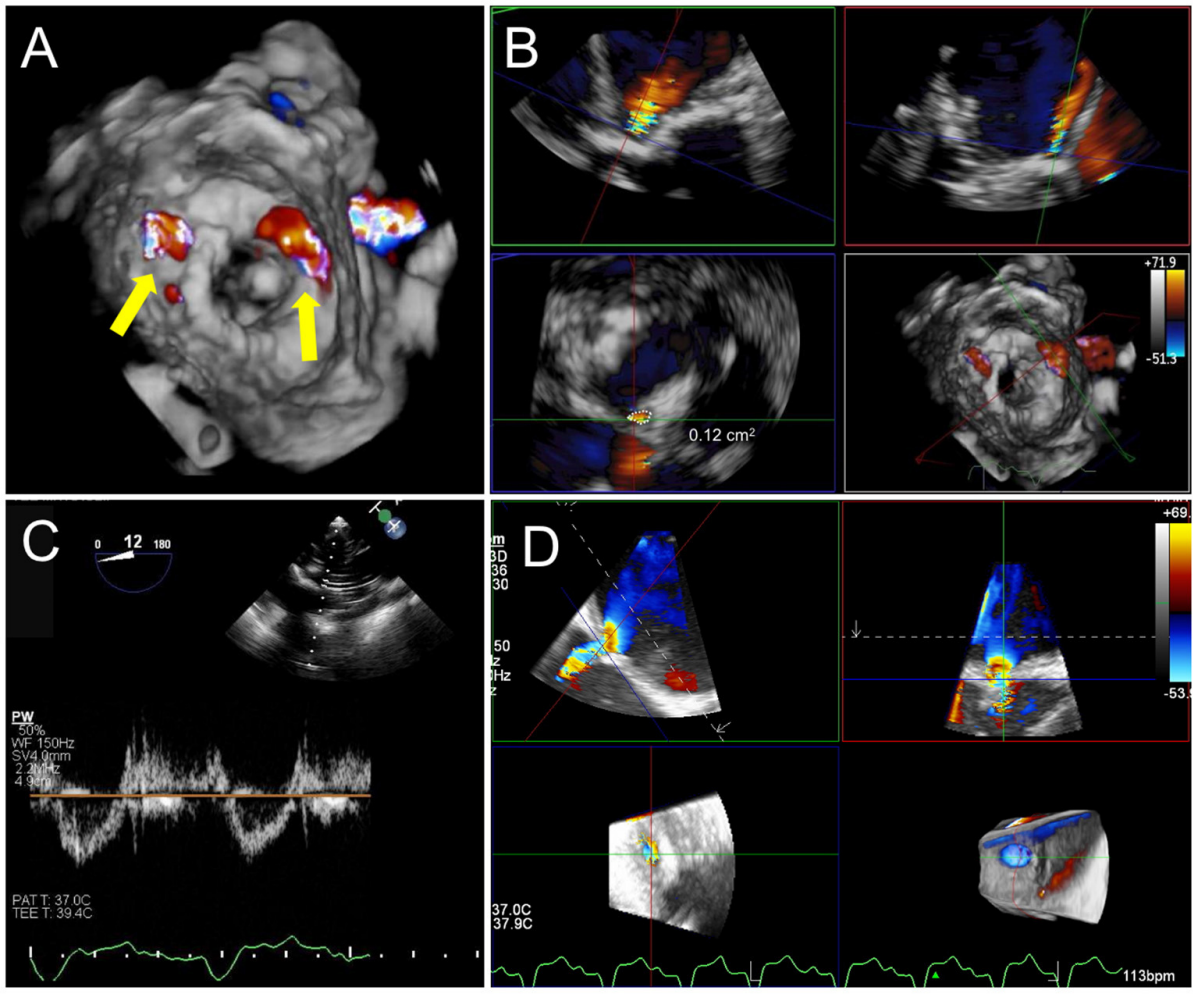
Post TMVR assessment immediately after deployment. (A) 3D color Doppler evaluation from an en-face surgeon's view immediately after a valve in MAC implantation reveals two areas of paravalvular leak (PVL) at 10 and 1 o'clock (yellow arrows). See Supplementary Video 3 for 3D en face evaluation of valve morphology and leaflet motion. (B) Careful interrogation of the largest area of PVL with 3D MPR reveals a vena contracta area of 0.12 cm2. (C) Pulsed-wave Doppler interrogation of the LVOT from a transgastric view in order to exclude a significant gradient or obstruction. (D) Evaluation of residual iatrogenic septal defect size and direction of flow.
